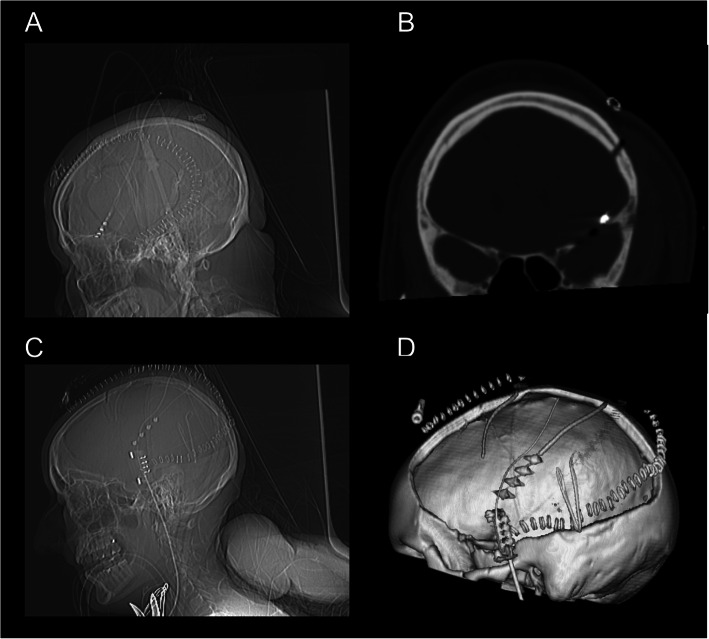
a Postoperative scout of CT scan with frontal EEG electrodes. b Coronary reconstruction of the CT scan. c Postoperative scout of CT scan with temporal EEG electrodes. d 3D reconstruction of the CT-scan
